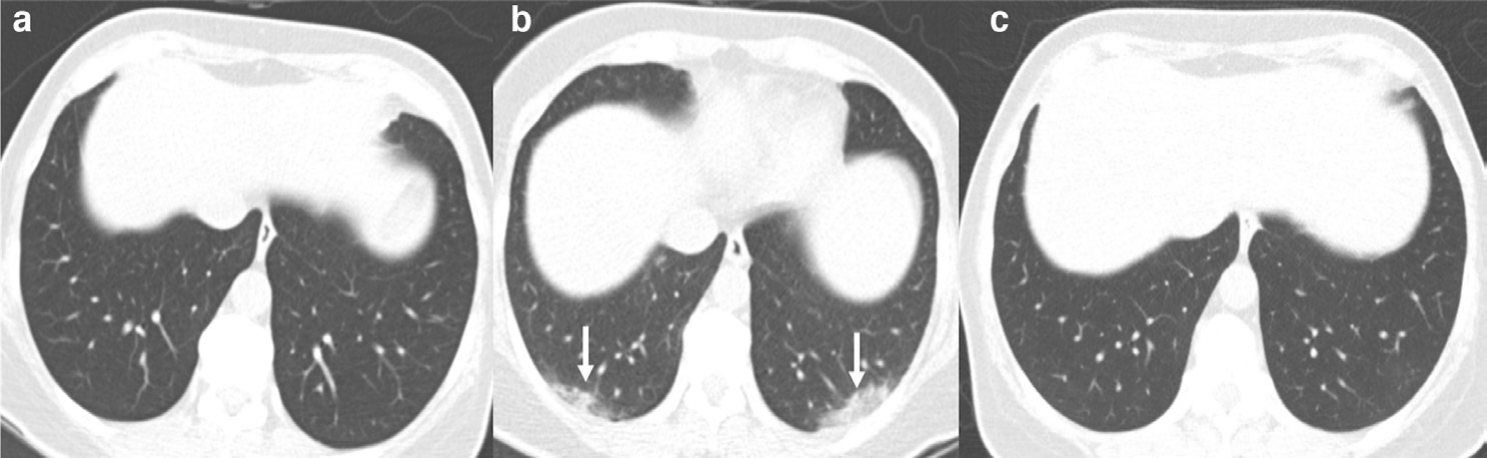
Longitudinal CT images in a patient with COVID-19 infection. Initial CT (A) obtained when the patient was symptomatic for 1 day showed no abnormality. Follow-up CT obtained on day 7 (panel-B) due to persistent symptoms showed peripheral bilateral lower lobe ground-glass opacity (arrows). CT obtained a month later (C) showed complete clearance of the disease.
